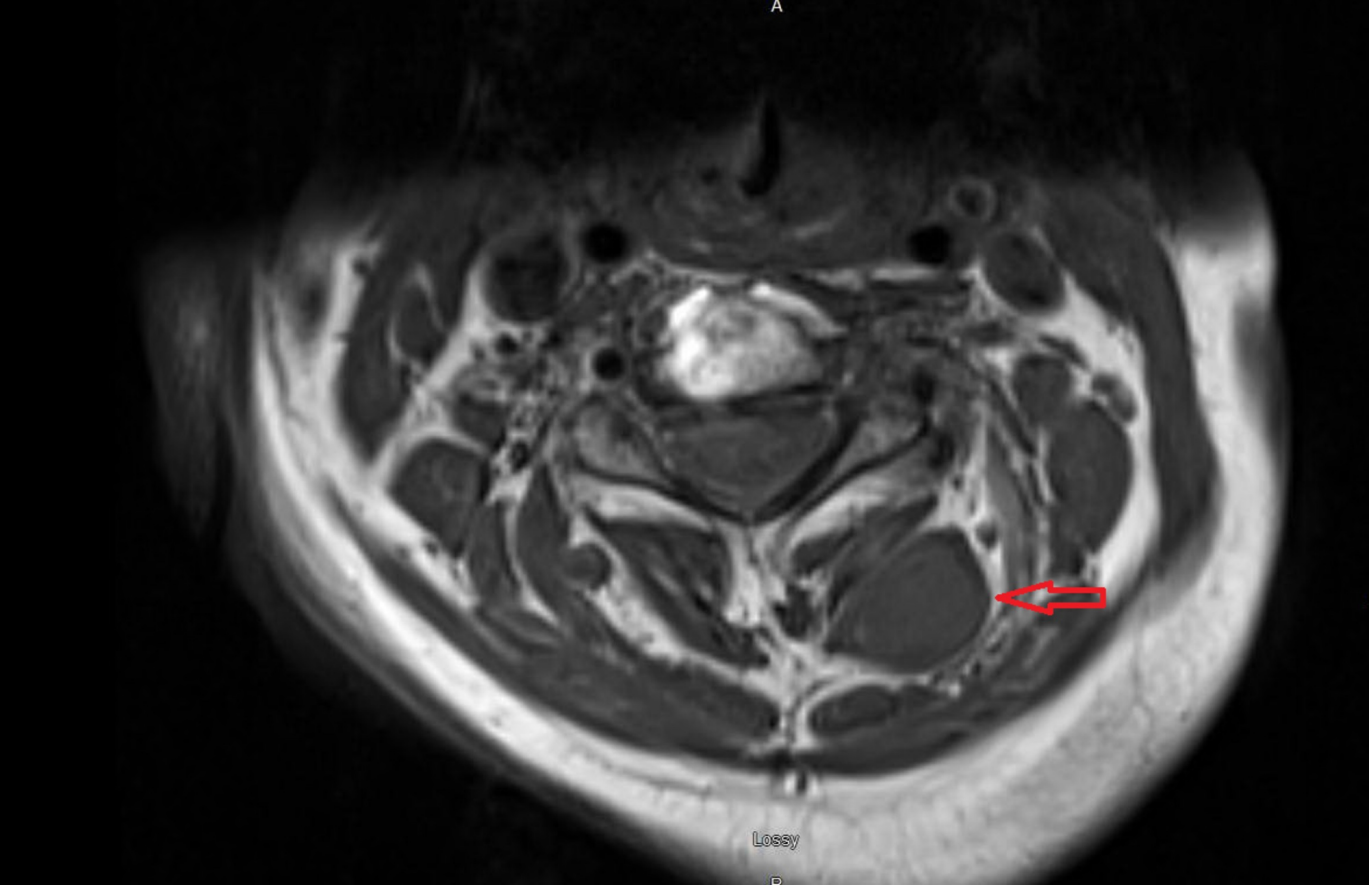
Axial MRI of lesion. T1 weighted MRI of cervical spine with axial images showing a hypointense mass in posterior elements from C2 to C4 measuring approximately 3.5 cm x 1.7 cm x 1.6 cm.
